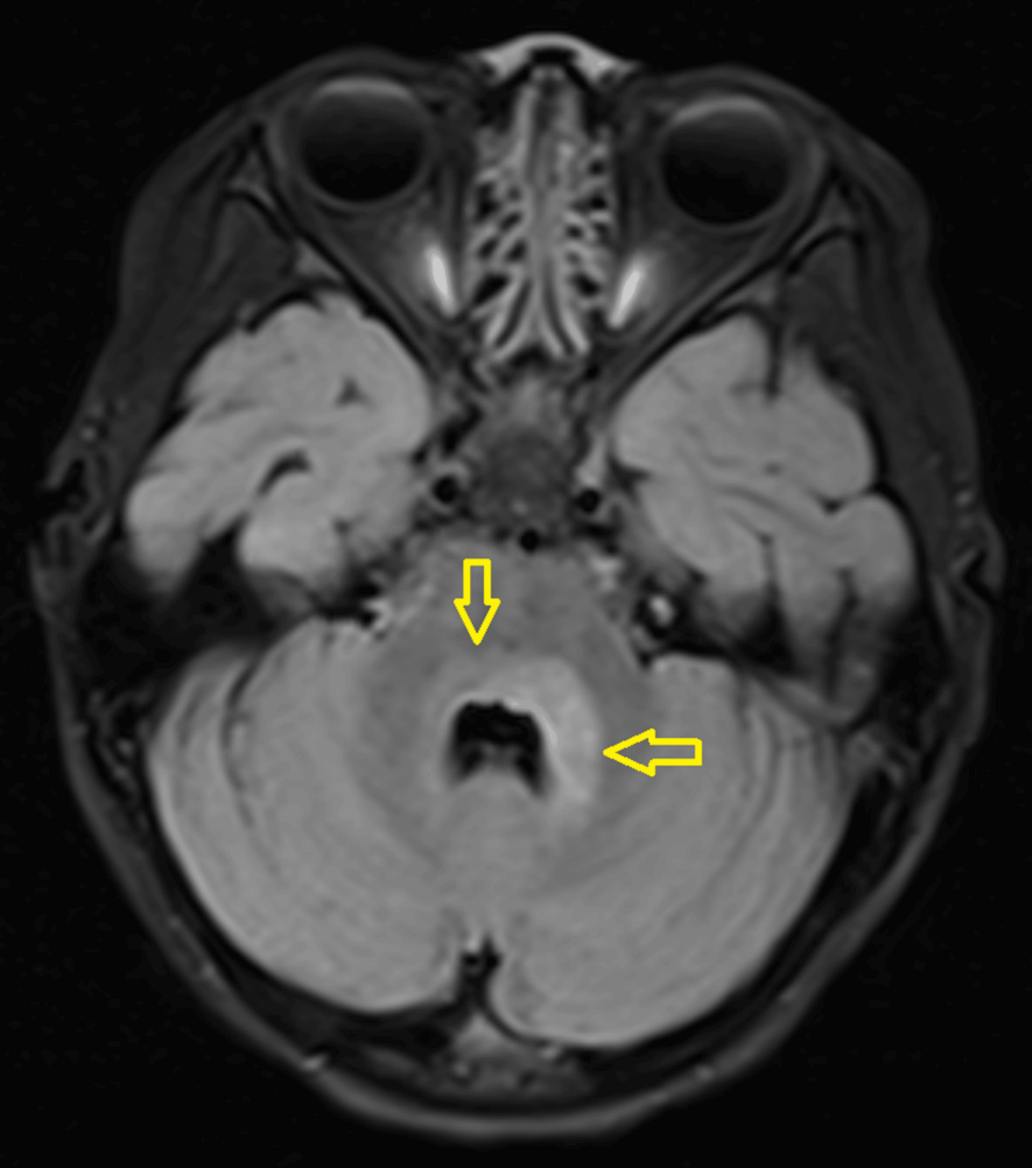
T2-Weighted Fluid-Attenuated Inversion Recovery (T2W/FLAIR) hyperintense white matter lesions noted at bilateral cerebral peduncles (yellow arrow).
